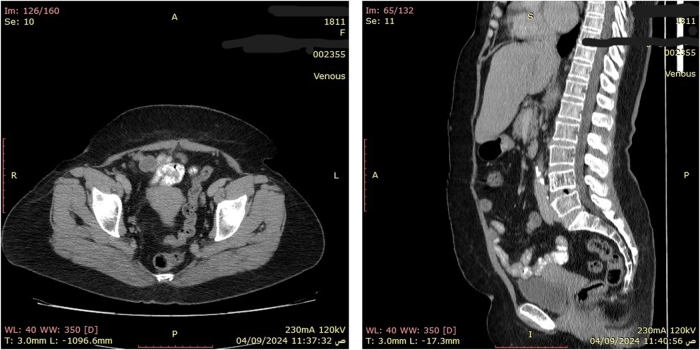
(A), (B) Follow-up contrast-enhanced CT scans at 6 months post-operatively showing no evidence of local recurrence or metastatic disease.
